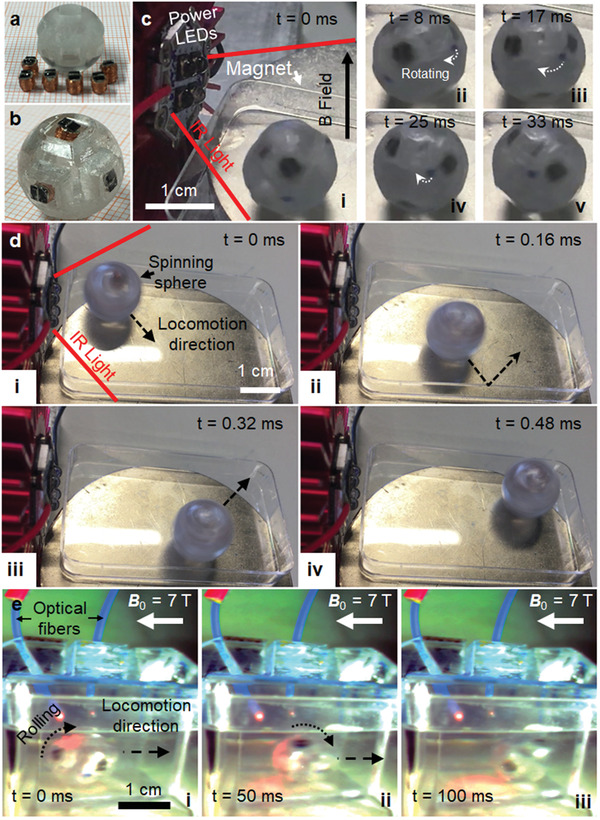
Characterization results of the wireless six‐module spherical actuator. a) Photograph of the 3D‐printed plastic sphere and six actuator modules before assembly. b) Photograph of the assembled spherical roller with six modules. c) Rotation of the spherical roller on a 0.25 T permanent magnet with the applied IR light at different time‐lapses. d) Motion of the spherical roller on a 0.25 T permanent magnet with IR light radiation as it spins and hits the walls of the plastic container. e) Rolling and forward motion of the sphere inside the preclinical MRI scanner (*B*_0_ = 7 T) inside a plastic container upon turning on IR light through two optical fibers.
